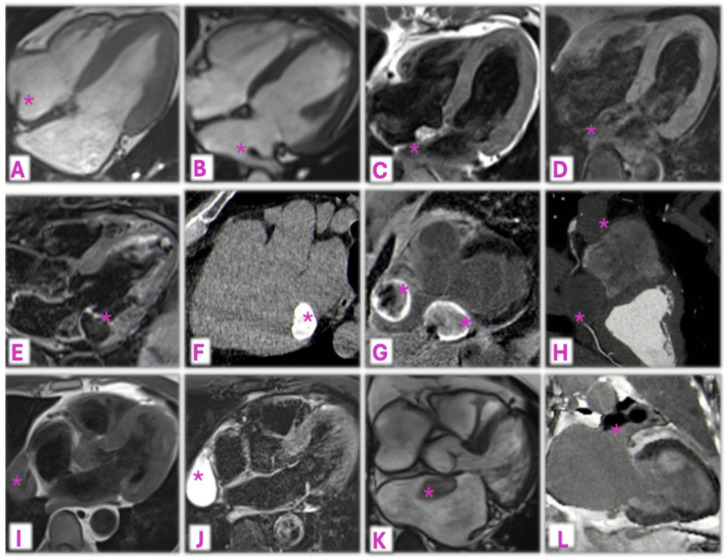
Examples of normal anatomical structures and non-tumoral masses at CMR/CCT (*). Panel (A): Crista terminalis; Panel (B): Coumadin ridge; Panels (C,D): Lipomatous hypertrophy of the interatrial septum showing hyperintense signal in turbo-spin-echo T1-weighted images (C) which notably decreases with fat saturation (D); Panels (E,F): Caseous mitral annular calcification has low signal on T2-weighted CMR images (E) and increased density on CCT (F); Panels (G,H): Two thrombosed aneurysms of the right coronary artery showing no-contrast Gadolinium uptake on CMR (G) and no iodine uptake on CCT (H); Panels (I,J): pleuro-pericardial cyst appearing homogeneously isointense in turbo-spin-echo T1 (I) and hyperintense in T2-weighted CMR images (J); Panels (K,L): examples of intracardiac thrombi, in the LA on cine-SSFP imaging (K) and in the LAA showing no-contrast uptake in LGE imaging (L).
